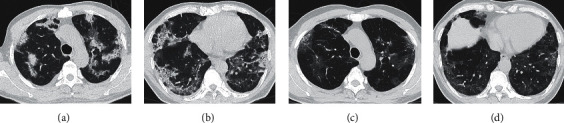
A 55-year-old male patient with a severe case of COVID-19. (a, b) Consolidations and ground-glass opacities were seen in multiple lobes and segments of both lungs on day 25 after onset of the disease. The density was not uniform, and there was an evidence of air bronchograms and intralobular septal thickening. (c, d) On day 47 after onset and day 21 after discharge, ground-glass opacities and fibrous strip shadows were seen in multiple lobes and segments of both lungs. Consolidations dissipated and the intralobular septal thickening was milder compared to the last CT of admission. The total volume of pulmonary lesions was measured and calculated through two CT scans, and the pulmonary lesions were dissipated to a slight degree.
